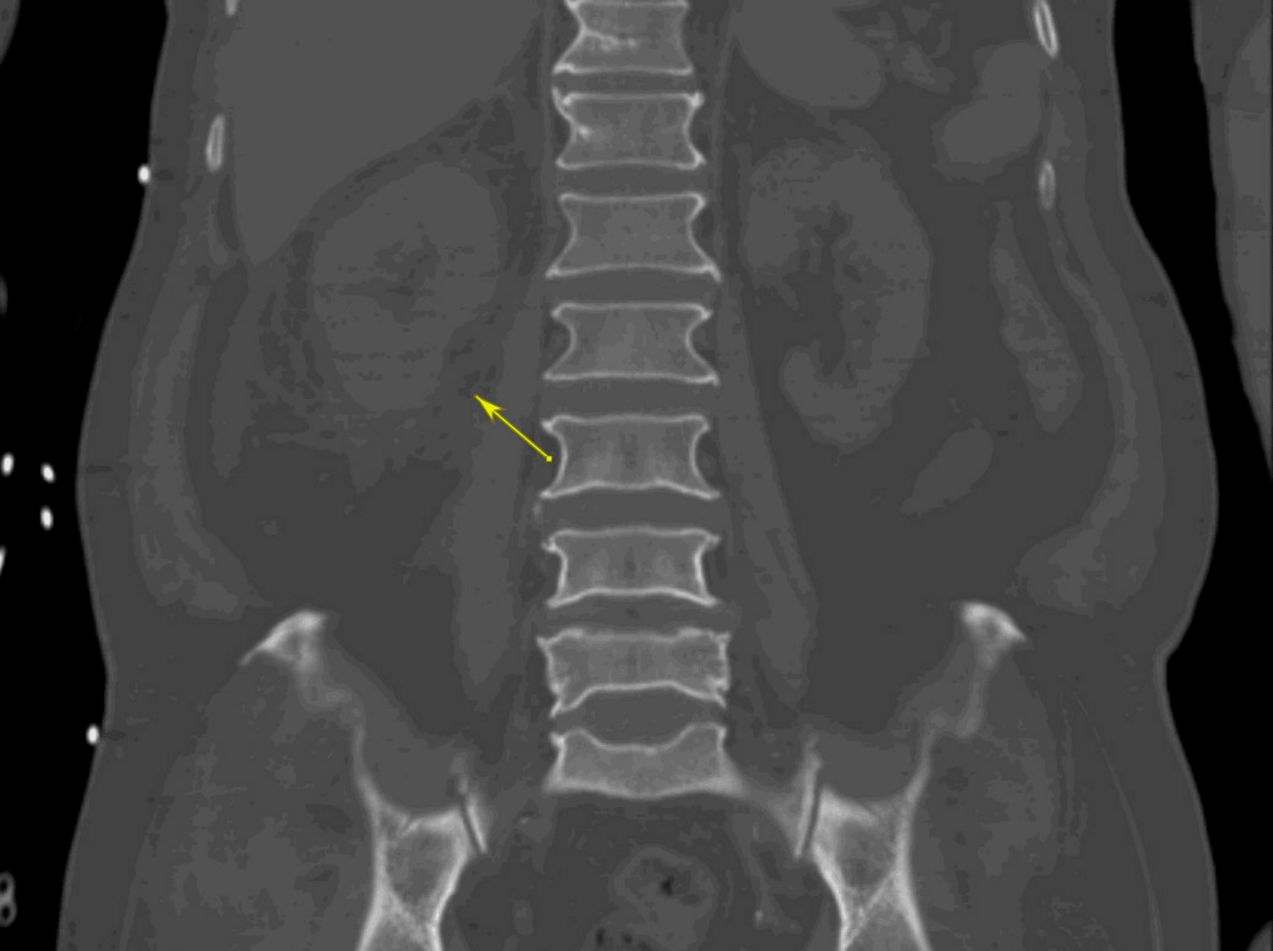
Coronal section of patient's CT scan of the abdomen and pelvis showing fat stranding around the right kidney (yellow arrow)
